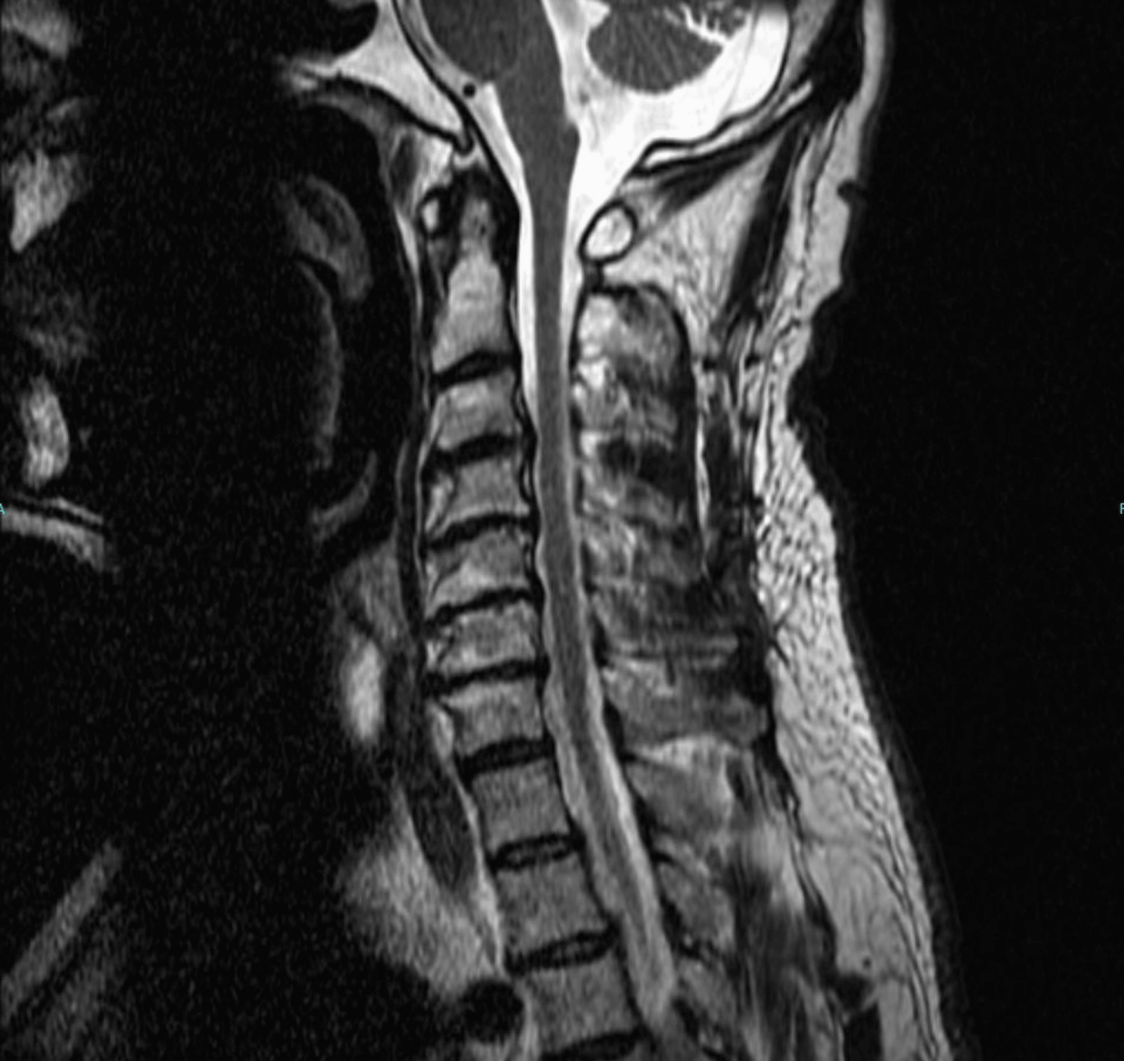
Case D: Post-surgical MRI. There is no evidence of spinal cord compression. There is mild nerve root impingement predominantly at C5-6 and C6-7 disc levels.
